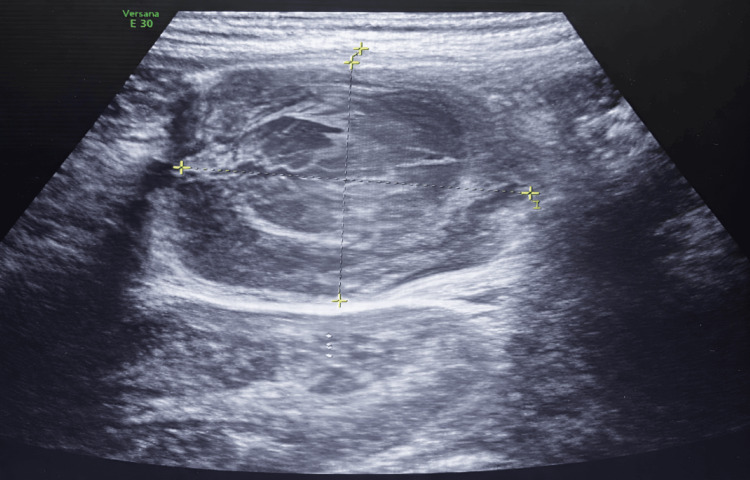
Abdominal ultrasound showing a right ileocecal mass with associated inflammatory changes
